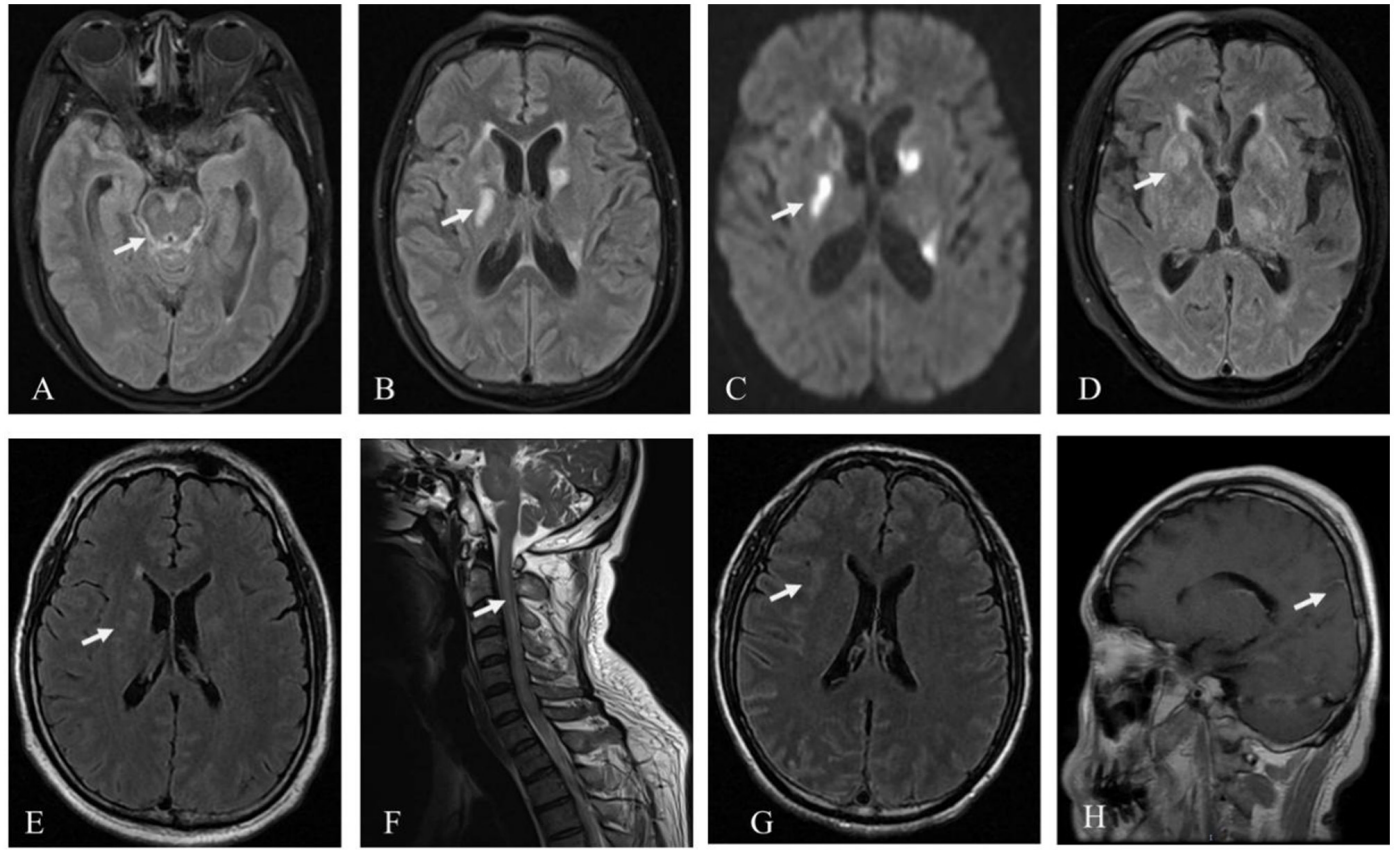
Patient MRI images. Hyperintensity signal inthe brainstem, pia mater, and basal ganglia on T2 FLAIR (A, B) and the basal ganglia on DWI in the patient in Case 2 (C); hyperintensity signal in the basal ganglia on T2 FLAIR in Case 3 (D); hyperintensity signal in the periventricular white matter on T2 FLAIR (E), and the cervical spinal cord on T2 in the patient in Case 4 (F); hyperintensity signal in the subcortex on T2 FLAIR and parieto-occipital sulci meningeal enhancement on T1 in the patient in Case 5 (G, H).
